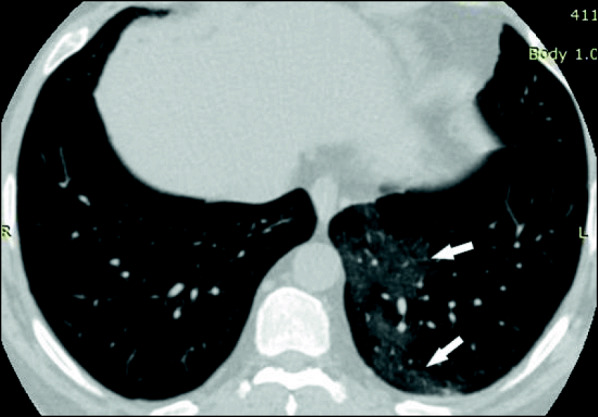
A 65-year-old man who presented with fever, headache, fatigue, and myalgias of 5 days duration. His PaO_2_/FiO_2_ ratio was 410 mmHg. Axial thin-section unenhanced CT image shows peripheral posterior ground-glass opacities in the left lower lobe with a rounded morphology (arrows)
